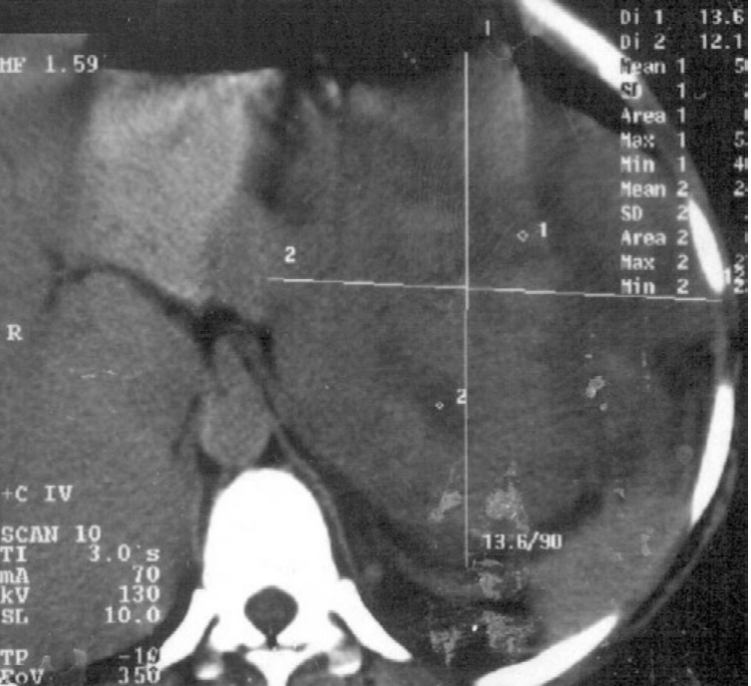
contrast enhanced CT abdomen of the patient showing the same lesion as in fig. 1 which is non enhancing.
